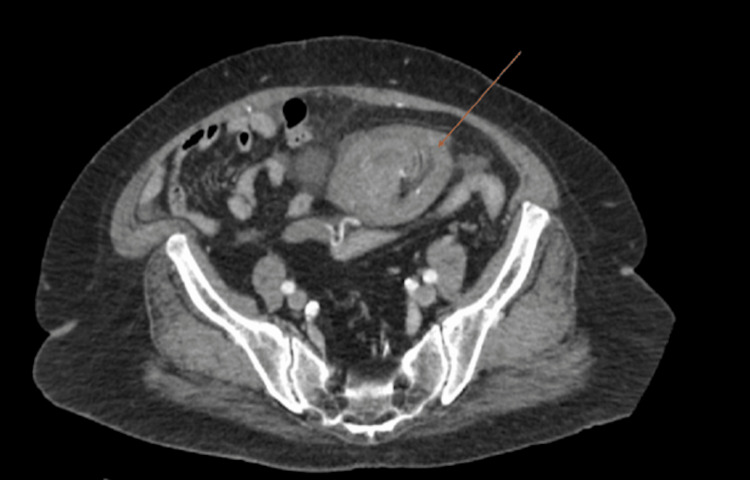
CT scan demonstrating whorled and twisted appearance of the cervix (indicated by an arrow)
